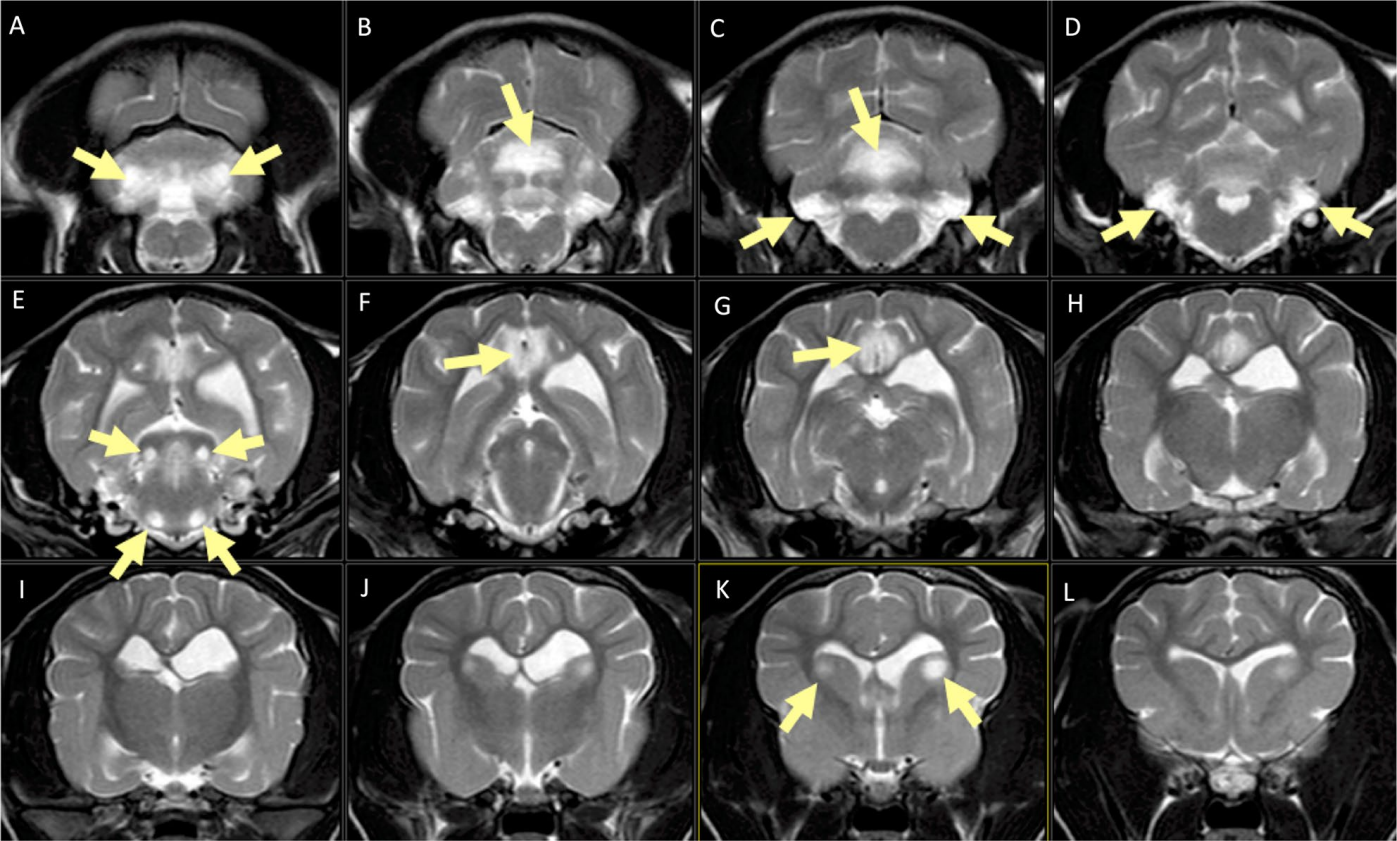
Transverse T2-weighted MRI images of case 2 from caudal to rostral, showing the bilateral and symmetrical T2-weighted hyperintense lesions. (A–D) Cerebellar cortex, white matter and cerebellar nuclei (arrows). (E) Rostral colliculi and brainstem nuclei (arrows). (F–H) Cingulate gyri (arrows). (J–L) Caudate nuclei.
